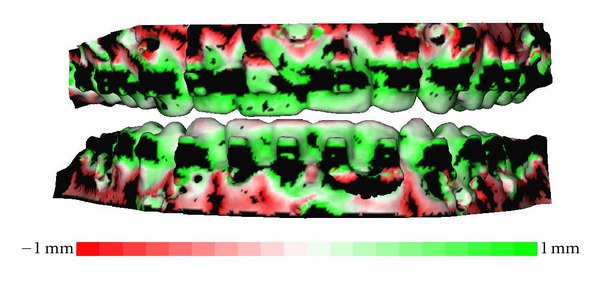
Distance map of the matched dentition of the CBCT of the patient and the impressions. It ranges from −1.0 mm to 1.0 mm.
